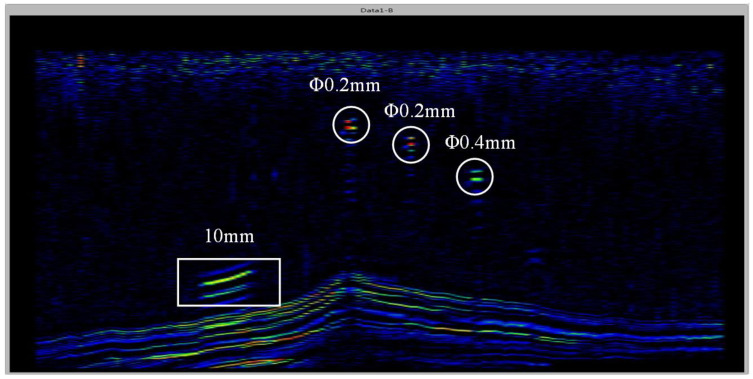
Ultrasonic B-scan images of inlet and exhaust edges of the blade.
